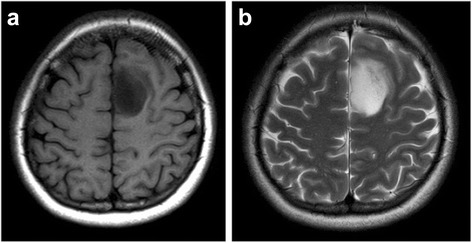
Axial sections of magnetic resonance imaging scan performed 3 days before operation. T1-weighted image as hypointense (a) and T2-weighted image as hyperintense (b); mass is located in the left superior frontal gyrus
